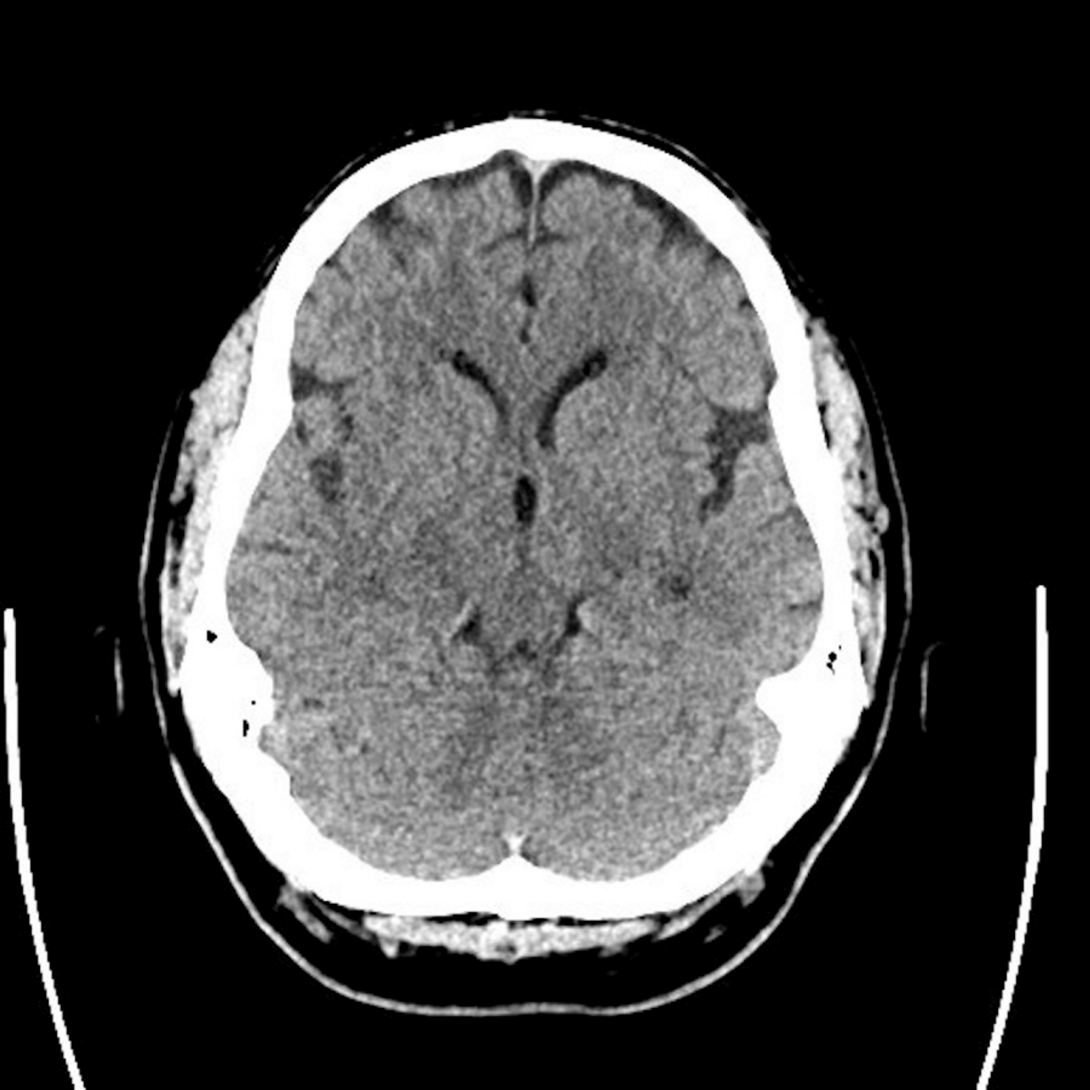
Unremarkable initial CT without contrast evaluation of the brain
